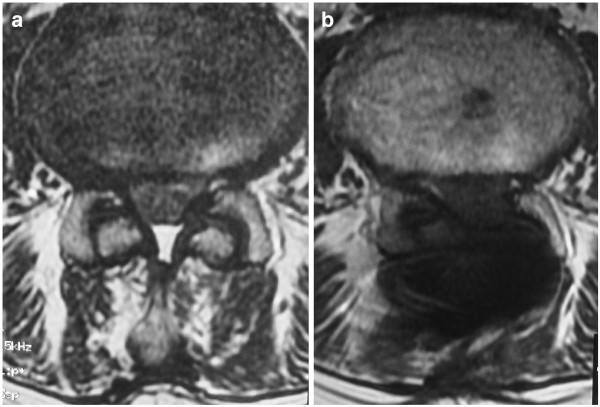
Preoperative (A) and postoperative (B) transverse T2 MRI images at the stenotic level of the same patient shown in Figure2. Unilateral microdecompression and X-STOP insertion was performed. The postoperative increase of the space available for the sac is shown.
